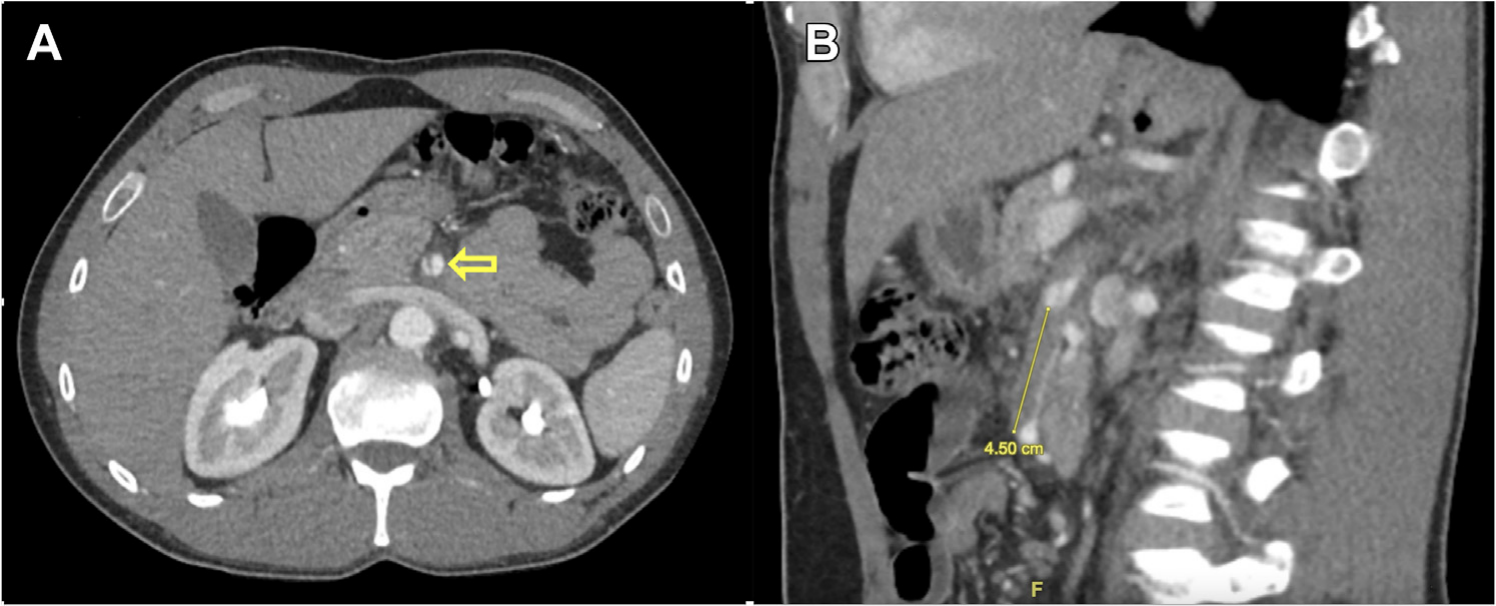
(A) Cross-sectional computed tomography angiography image of the abdomen shows proximal superior mesenteric artery dissection with an arrow indicating the intimal lining flap. (B) Coronal section of computed tomography angiography image of the abdomen shows superior mesenteric artery dissection with associated 1.3 x 4.5 cm thrombosis, causing 80% stenosis of the arterial lumen.
